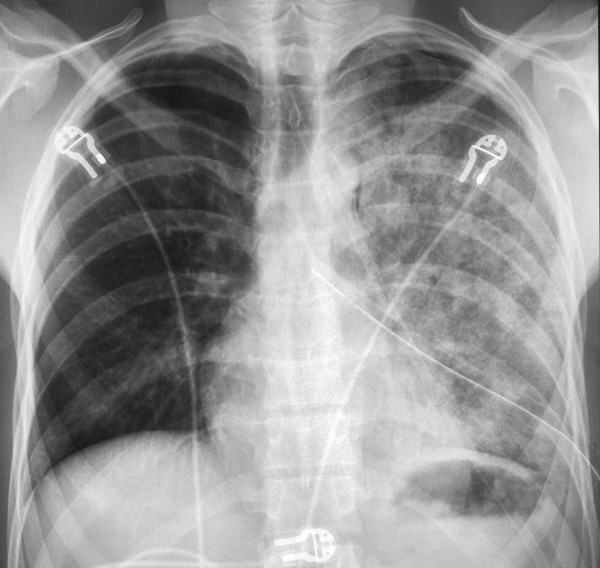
Chest radiography with left-sided pulmonary edema.
